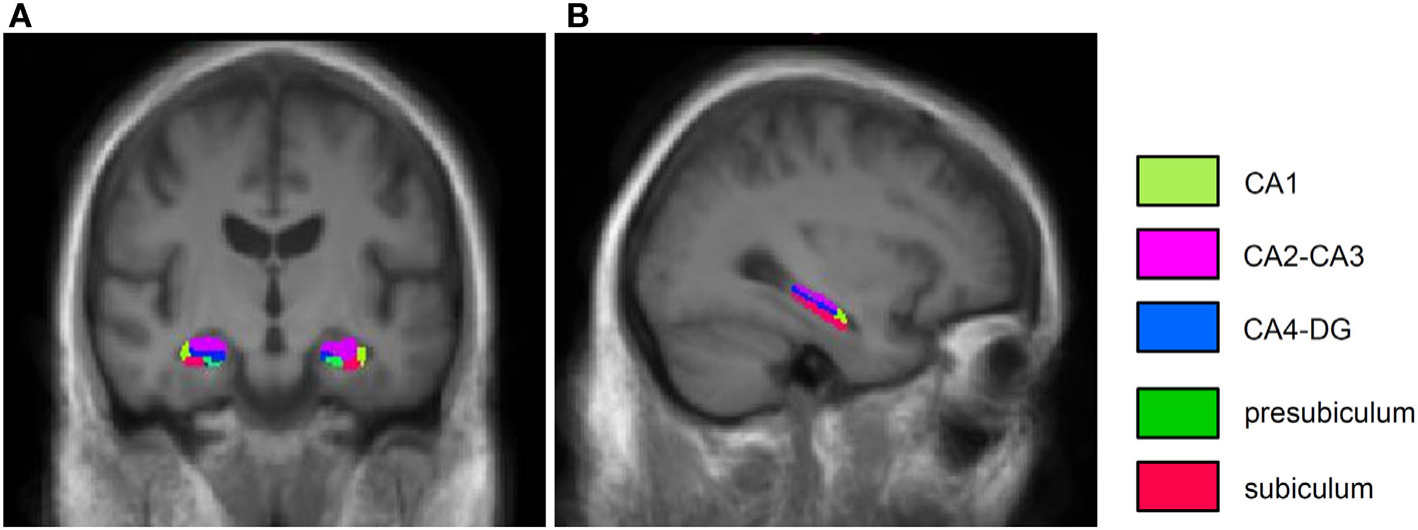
Coronal (A) and sagittal (B) T1-weighted images from the average output of FreeSurfer hippocampal segmentation from healthy individuals.
